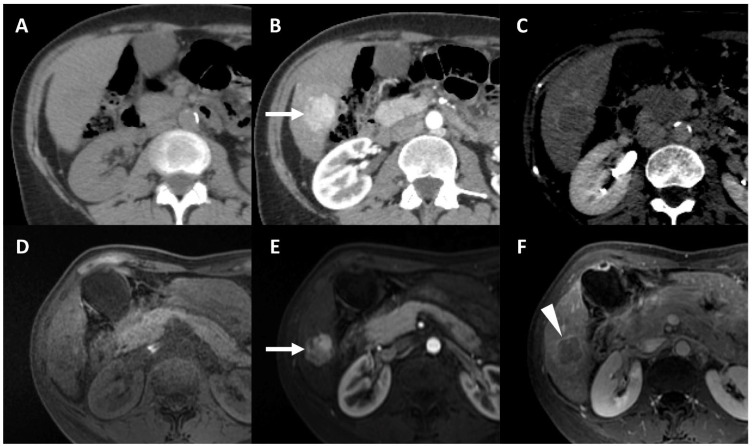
Typical appearance of hepatocellular carcinoma (HCC) on both contrast enhanced CT (A–C) and MRI (D–F) in the segment VI of the liver in a patient with hepatitis C-related liver cirrhosis. At unenhanced CT (A), the lesion is isoattenuating, while it is slightly hypointense on unenhanced T1 fat-saturated MR image (D), compared to the background liver. The lesion shows nonrim arterial phase hyperenhancement on both CT ((B) arrow) and MRI ((E), arrow) and washout on delayed venous phases on both CT (C) and MRI (F). Note the enhancing capsule which is best depicted on delayed phase at MRI ((F) arrowhead).
